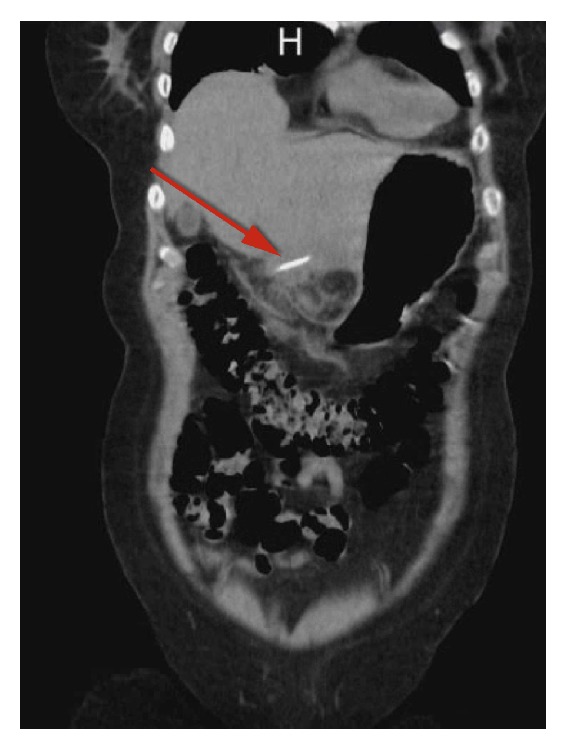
Coronal view CT scan of the abdomen and pelvis showing radiopaque FB in the liver (red arrow).
